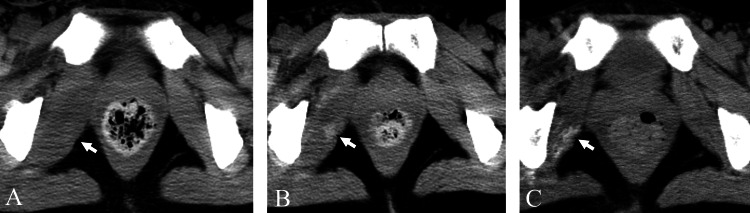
Case five A 41-year-old woman with myositis ossificans in the right short external rotator muscles. (A) On the 20th day of hospitalization, CT showed swelling of the right muscles (arrow). Manual muscle testing score in the right lower limb was 2. No other source of fever was identified. (B) On the 23rd day, CT revealed calcification within the swollen muscles (arrow). (C) On the 49th day, follow-up CT demonstrated ossification with a decrease in swelling (arrow).
